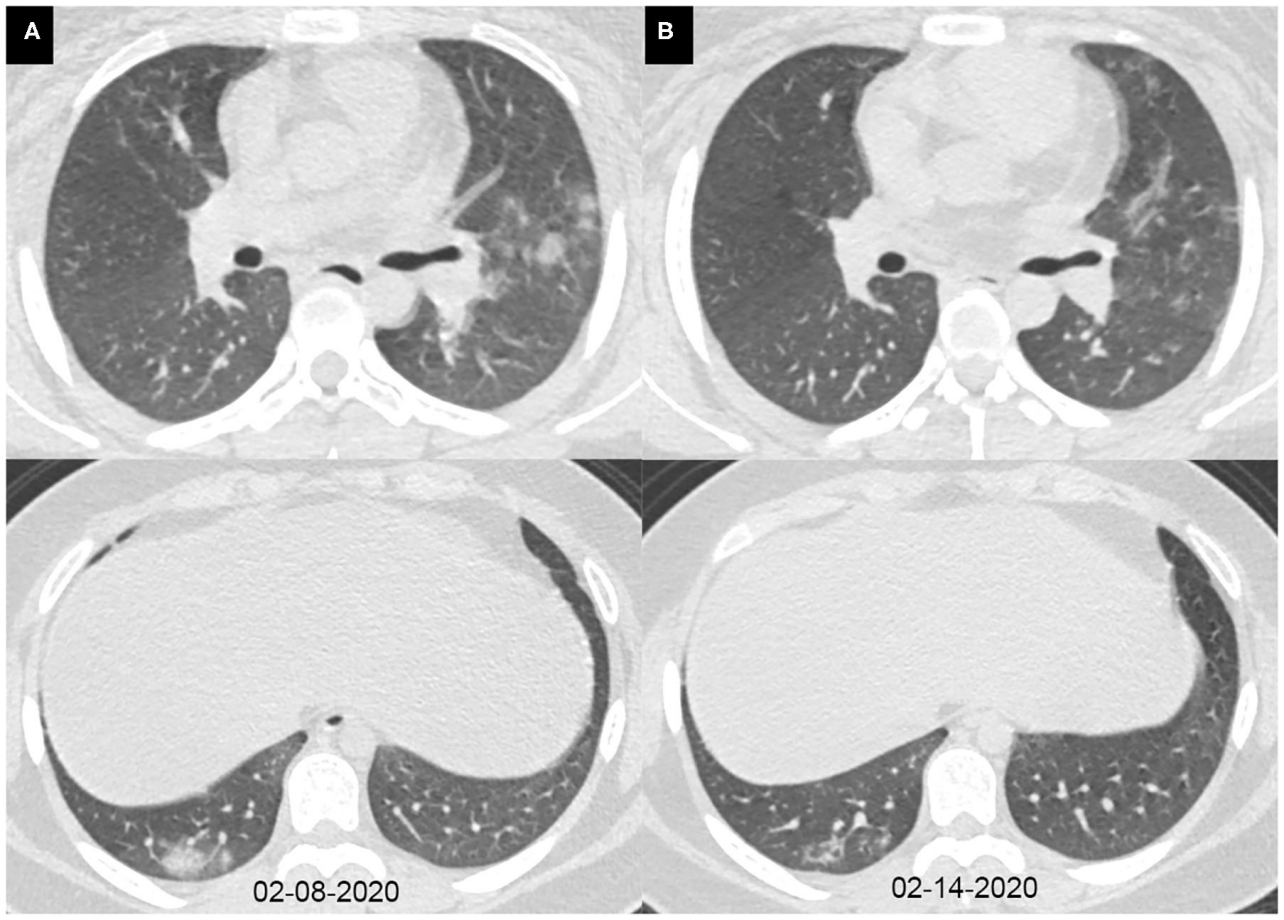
Axial chest CT images of a 15-year-old girl with fever, cough, diarrhea, and headache for 7 days. Pharyngeal swab RT-PCR tests were performed on February 12, 2020, with positive results, and on February 15 and 17, 2020, with negative results. Chest CTs were obtained on February 8 (A) and 14, 2020 (B). (A) The subpleural consolidations and nodules were seen in the right lower lobe and the left upper lobe. (B) The consolidations and nodules were obviously absorbed.
